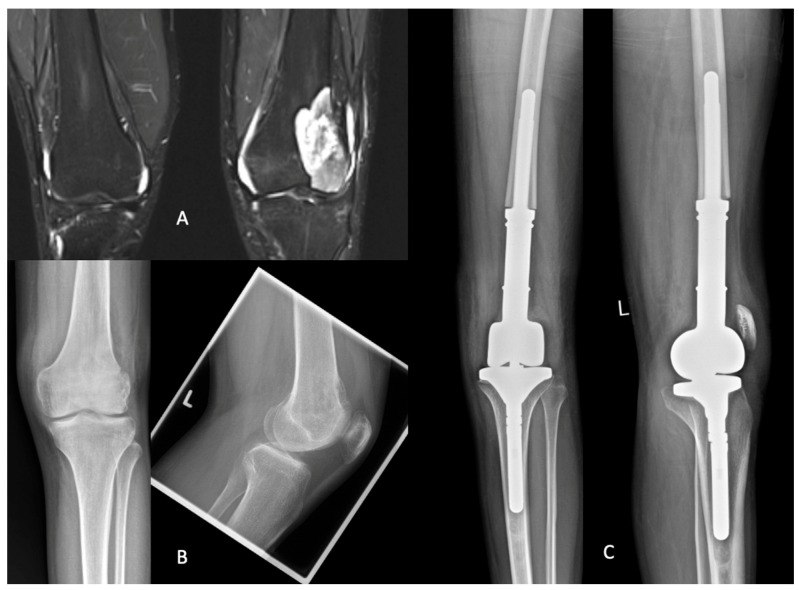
Radiological data of the described patient: (A) shows the osteosarcoma in the coronal plane of an MRI; (B) shows the osteosarcoma in an X-ray image in two planes; (C) shows the postoperative findings two years after the implantation of a tumor prosthesis.
